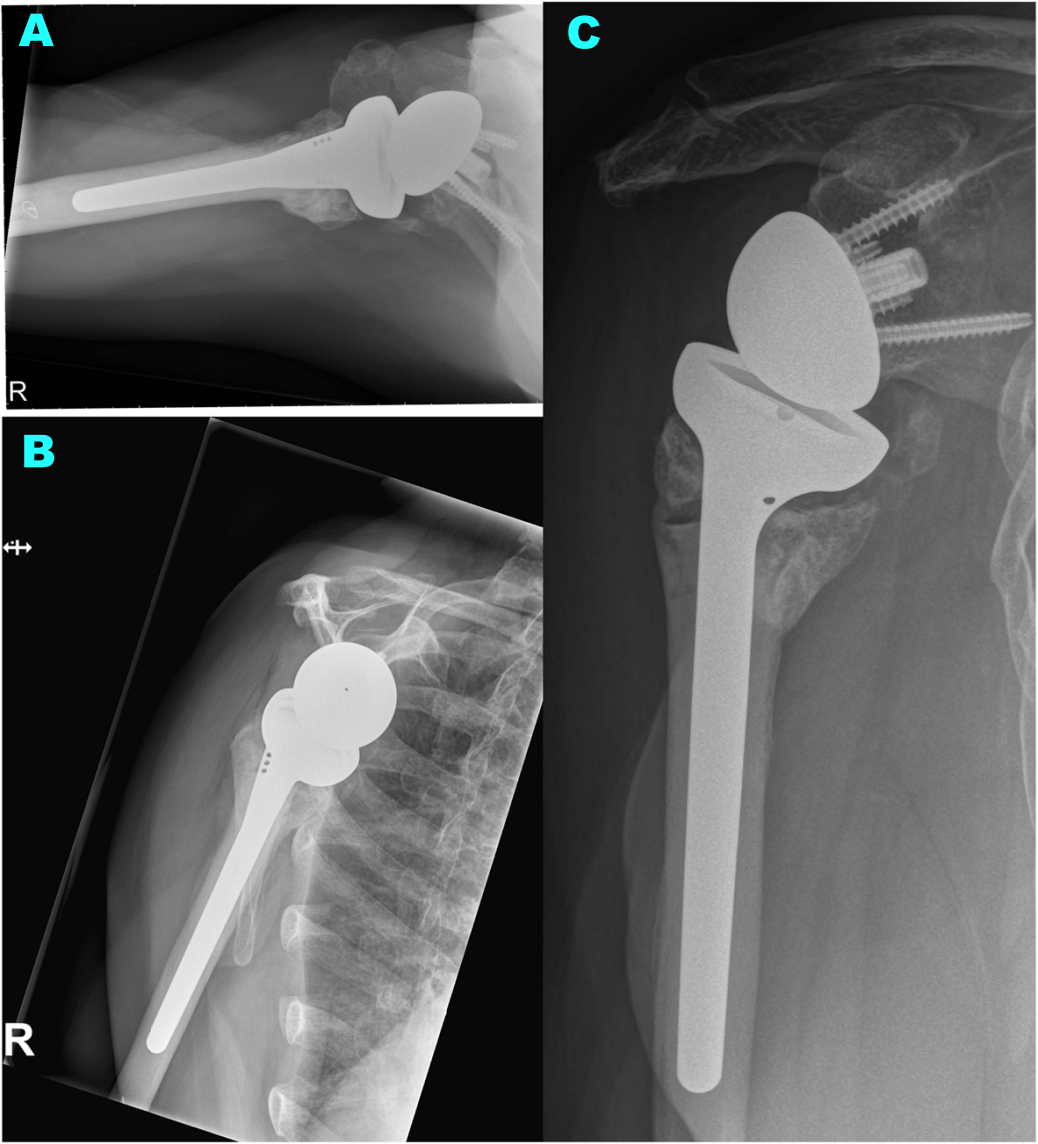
Anatomic healing of the tuberosities: A case of a 73 years old woman, an X-ray is pictured in three planes 60 months post operation. (a: axial view, b: supraspinatus outlet view, c: anterior-posterior view)
